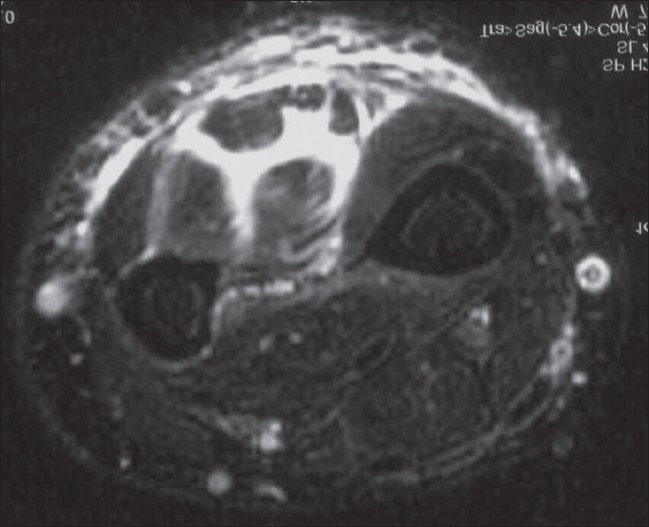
Tuberculous myositis of the forearm. Axial, fat-suppressed, T2W MRI shows high signal intensity within the muscle bellies as well as between the various flexor muscles. There is also increased T2 signal within the adjacent subcutaneous tissue
